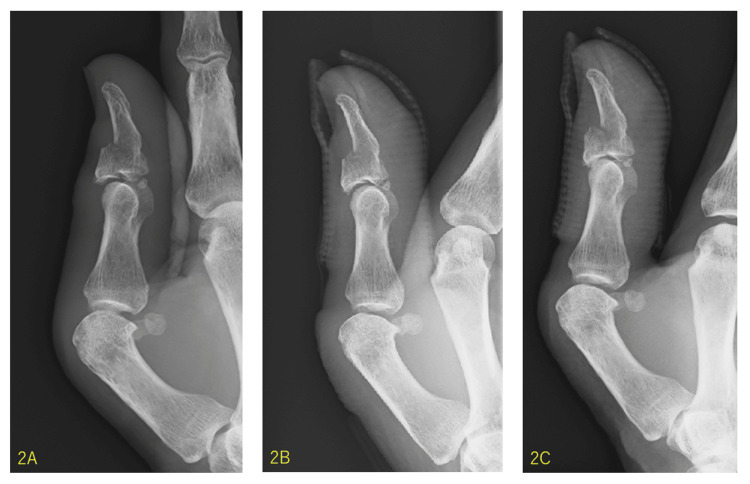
X-ray radiographs before operation. The X-ray radiography at the time of injury showed an intra-articular fracture at approximately 40% of the dorsal side of the base of the distal phalanx, with mild transposition (2A). In the X-ray radiography after the non-invasive reduction, the dorsal fragment was reduced non-invasively under the X-ray fluoroscope (2B). In the X-ray radiography two weeks after the non-invasive reduction, the dorsal fragment dislocated again (2C).
